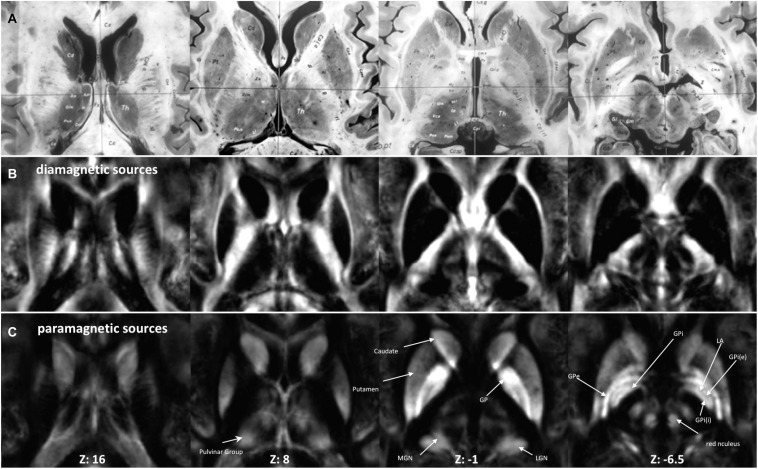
Anatomy and MRI of thalamus (Th) and basal ganglia (BG): (A) four selected slices were adapted (Schaltenbrand and Bailey, 1959) (B,C) Corresponding QSM images of diamagnetic and paramagnetic sources. GPe, external segments of the globus pallidus; GPi, internal segments of the globus pallidus; GPi (e), subsegment of internal segments of the globus pallidus; GPi (i), subsegment of internal segments of the globus pallidus; LGN, lateral geniculate nucleus; MGN, medial geniculate nucleus; LA, lamina accessoria.
